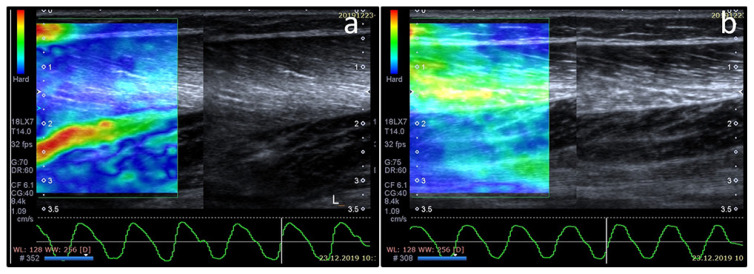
8-year-old male with inability to walk, flu-like symptoms, and increased serum CK level. Strain elastography of the symptomatic right side reveals an elastography score of 5 (a), while contralateral side has a score of 3 (b). Also note the increase in muscle thickness and echogenicity on the gray scale ultrasound on the symptomatic right side when compared to contralateral.
